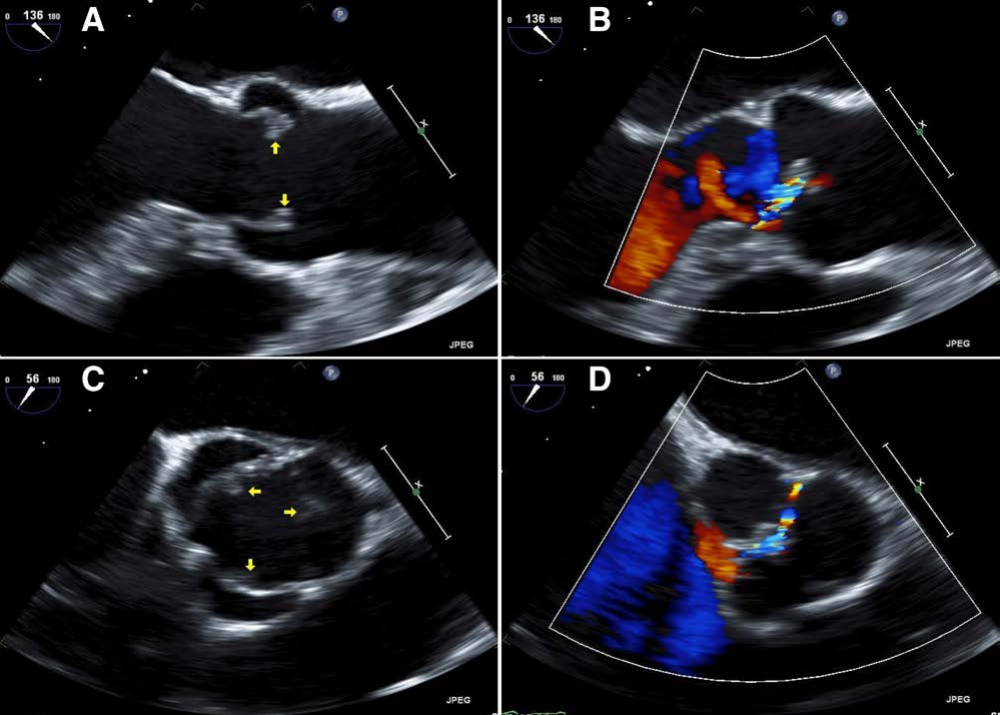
Transthoracic and transesophageal echocardiography. A mass adhering to the 3 leaflets is observed at the junction of the aortic valve.
